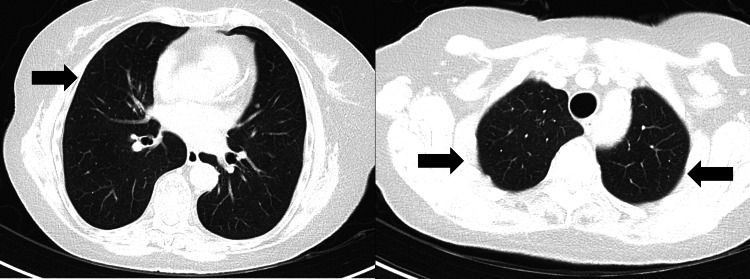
Thoracic CT scan 10 years after the first episode of radiation-induced organizing pneumonia. Presence of a few millimetric ground glass opacities (black arrows) surrounded by normal lung tissue.
